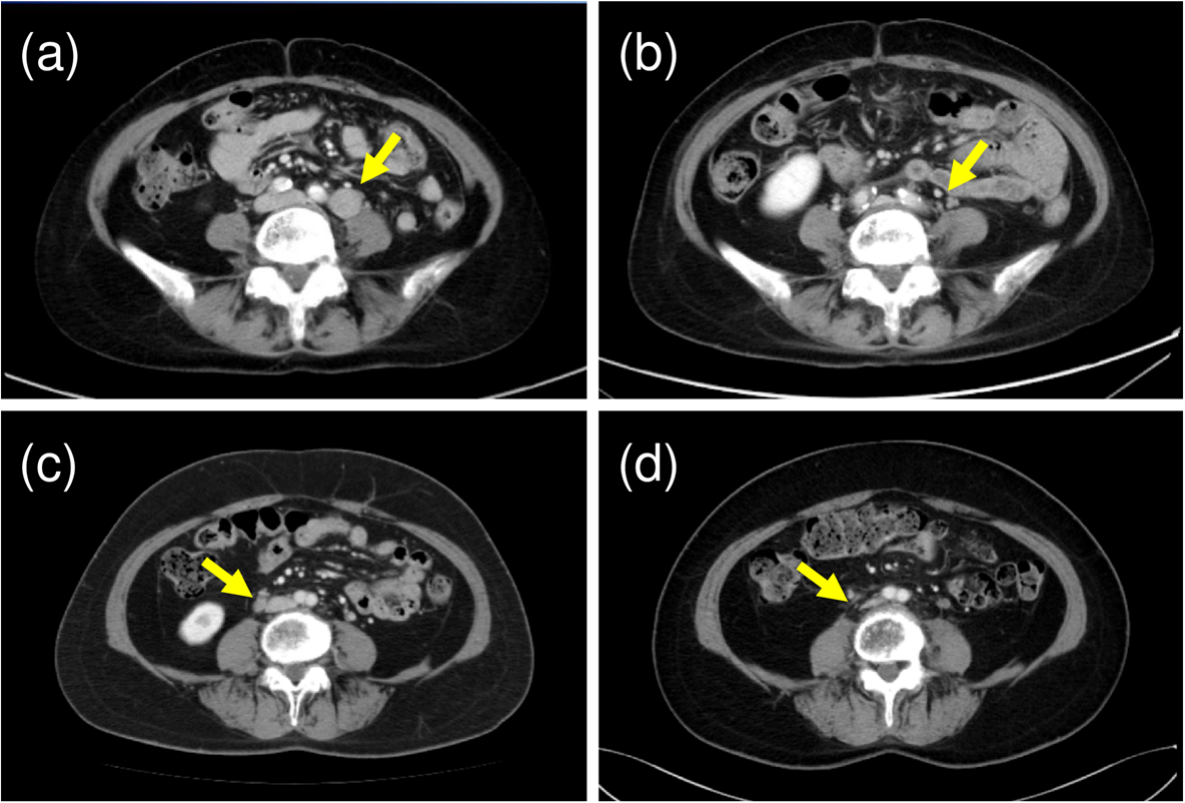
CT images of the recurred lymph nodes (LNs) at the time of recurrence ((a) Patient 1, (c) Patient 2)) and 3 years after carbon-ion radiotherapy ((b) Patient 1, (d) Patient 2). The recurrent LNs have disappeared after the therapy in both patients.
